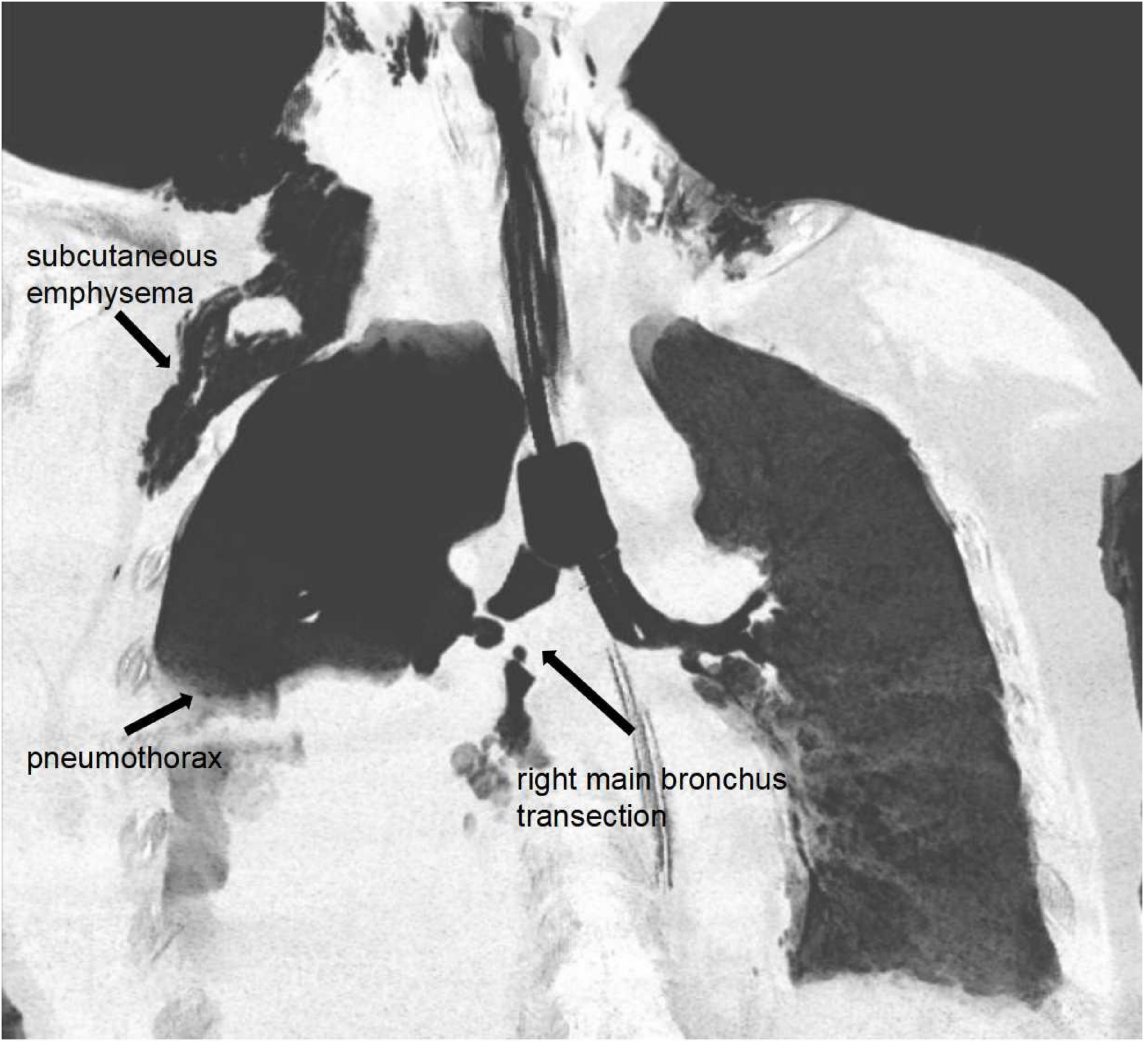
Chest computed tomography revealing complete transection of the right main bronchus with associated pneumothorax and mediastinal emphysema.
